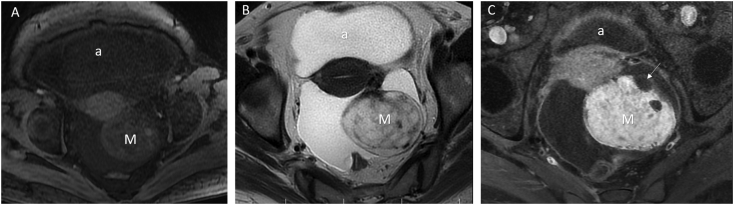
(A) Axial T1-weighted showed a well-defined heterogeneous hypo-to isointense solid mass in left ovary. (B) In axial T2-weighted mass appeared hyper intense and contains multiple thick septa. (C) Post contrast T1-weighted fat-suppression manifested avid enhancement in solid component. a = ascites, M = mass, Arrow = follicule.
